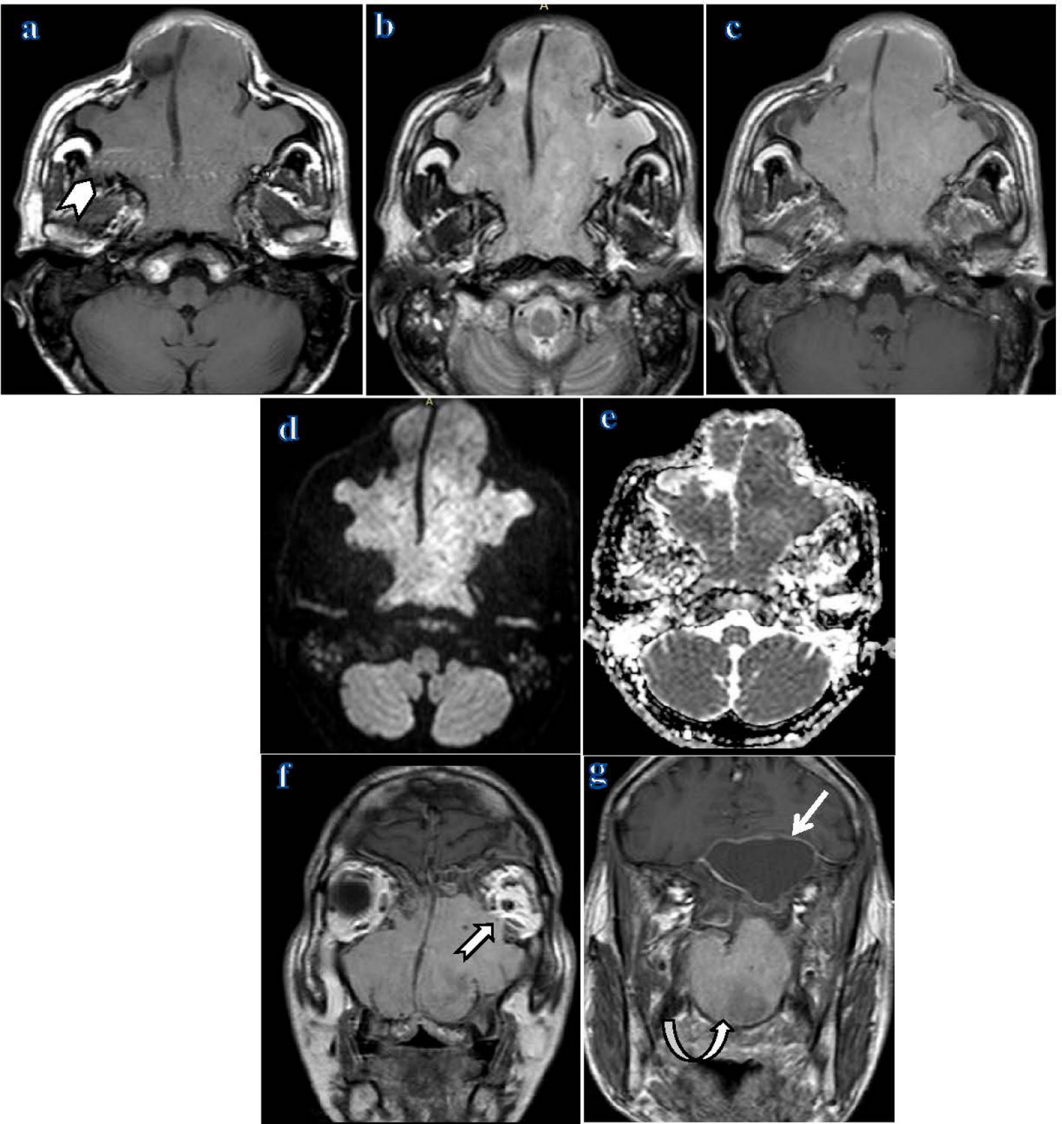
Contrast-enhanced MRI examination of the paranasal sinuses; (a) The axial *T*
